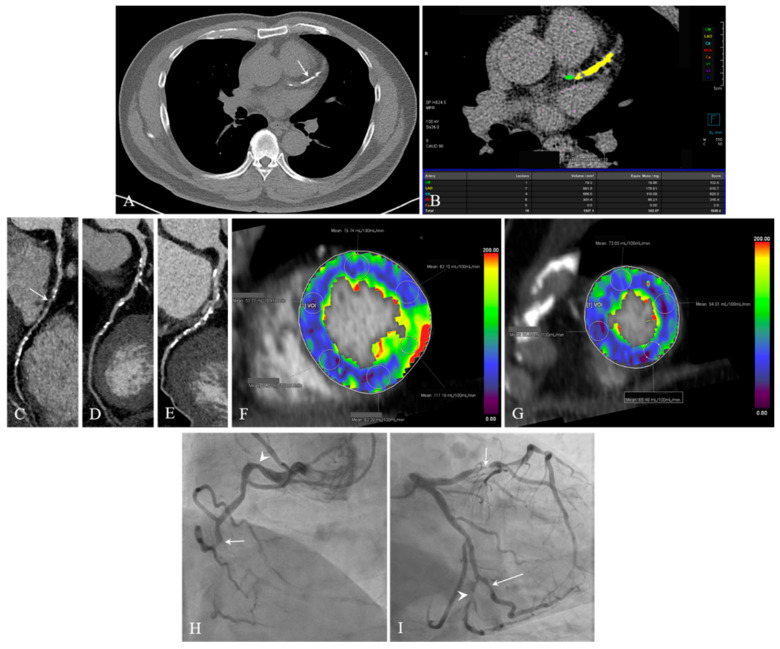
Images of a 60-year-old man with atypical chest pain. (A) Low-dose chest CT taken 45 days before CCTA shows severe CAC in the LAD (arrow). (B) Coronary calcium scan shows a high coronary calcium score of 1848.4 AU. (C–E) Curved MPR CCTA images of RCA (C), LAD (D), and LCx (E) show severe CAC. A severe stenosis with mixed calcified and noncalcified plaques is seen in the mid segment of the RCA (C, arrow). (F,G) CT-MPIs show an overall decrease in MBF from the mid- (F) to apical (G) segments, excluding the mid-inferolateral segment. (H,I) ICA identifies chronic total occlusion of the mid-RCA (H, arrow), significant stenosis (80–90% lumen reduction) in the proximal to mid-LAD (I, short arrow) and distal LCx (I, arrowhead), and significant stenosis (50–60% lumen reduction) in the proximal RCA (H, arrowhead) and secondary OM branch (I, long arrow). CT = computed tomography; RCA: right coronary artery, LAD = left anterior descending coronary artery, LCx = left circumflex artery, OM = obtuse marginal, AU = Agatston unit, MBF = myocardial blood flow, ICA = invasive coronary angiography, CT-MPI = computed tomography-myocardial perfusion imaging, CCTA = coronary computed tomography angiography, MPR = multiplanar reformation.
